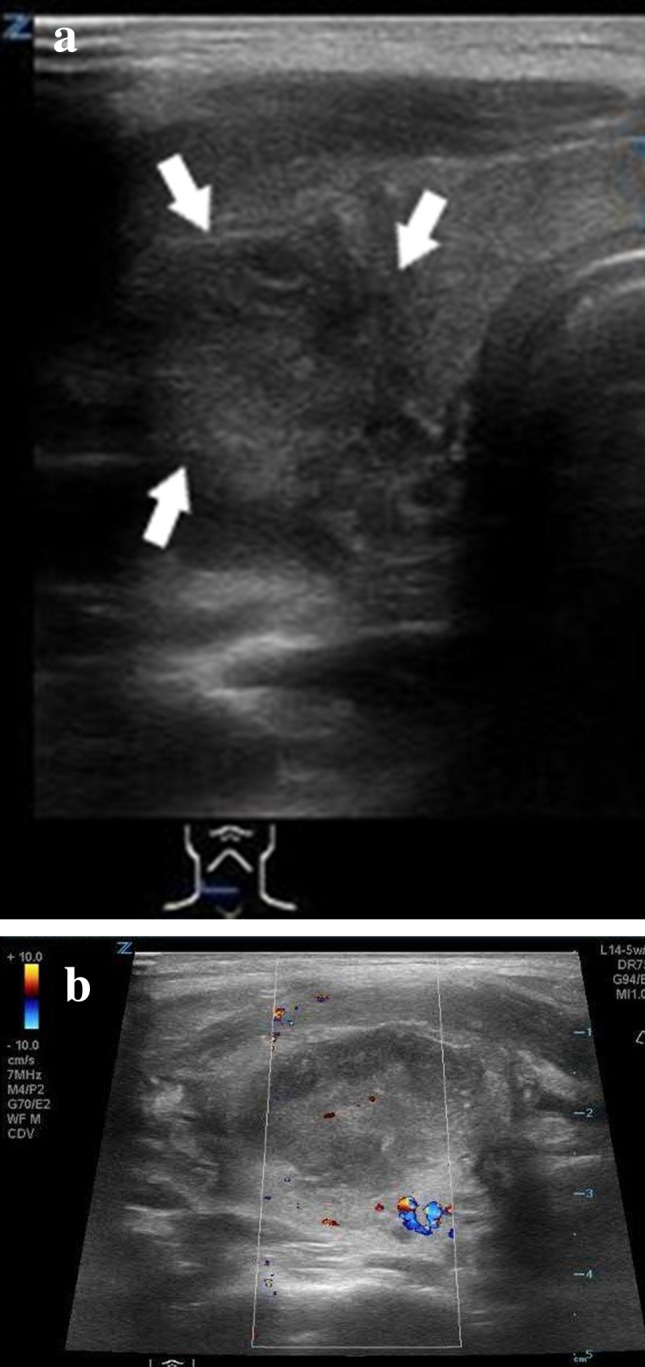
Conventional US and color Doppler US images in a 18-year-old male suffering from local pain, difficulties swallowing, fever. a B-mode US image in a transverse plane shows enlarged hypoechoic right lobe of the thyroid gland (arrows) in a patient with subacute non-suppurative thyroiditis. b Color Doppler US image in a longitudinal plane shows no vascular flow in a patient with subacute non-suppurative thyroiditis
